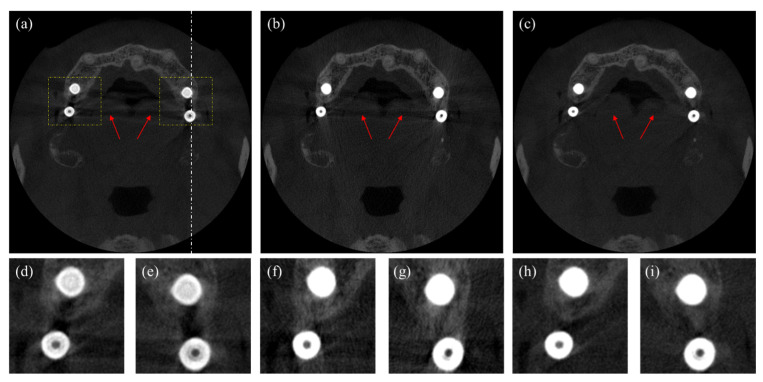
Axial images of a patient having four dental implants. (a) The uncorrected CT image, (b) the CT image corrected by the DSC method, and (c) the CT image corrected by the proposed method. The magnified images of the ROIs in (d,e) Figure 10a, (f,g) Figure 10b, and (h,i) Figure 10c. (Contrast window is [−1117~8564] in Hounsfield Unit).
